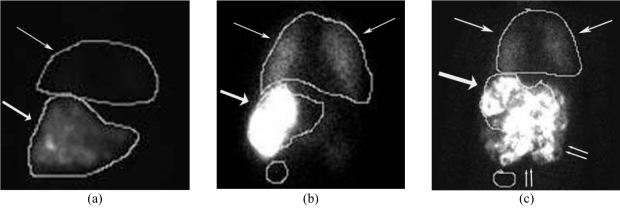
Planar nuclear medicine scintigraphy. Patterns of distribution of Tc99m MAA following hepatic arterial delivery (bold arrow-liver; thin arrow- lung; double arrows- gut). (a) Normal distribution with no extrahepatic activity and no significant hepato-pulmonary shunting. (b) Excessive hepato-pulmonary shunting. A 26% shunt fraction precluded treatment in this patient with hepatocellular carcinoma. (c) Significant gastrointestinal reflux. Treatment is precluded if this phenomenon is not corrected. Incidentally is also noted of excessive hepatopulmonary shunting.
